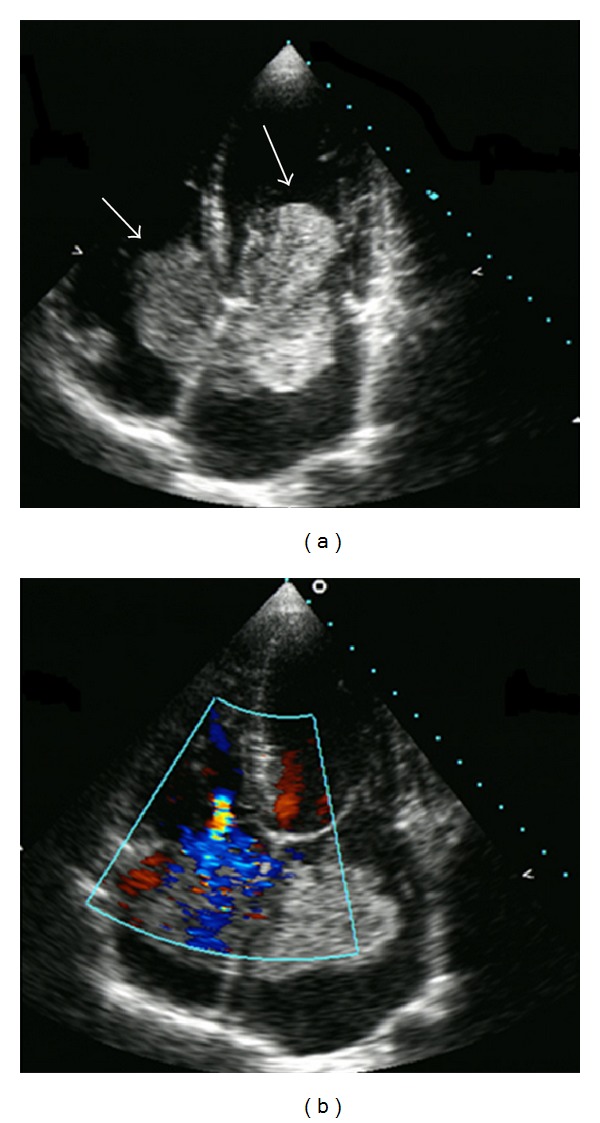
Echocardiogram showing bilateral atrial tumors (arrows), large left atrial mass protruding through the mitral valve during diastole. There was a mild tricuspid regurgitation on the color Doppler examination.
